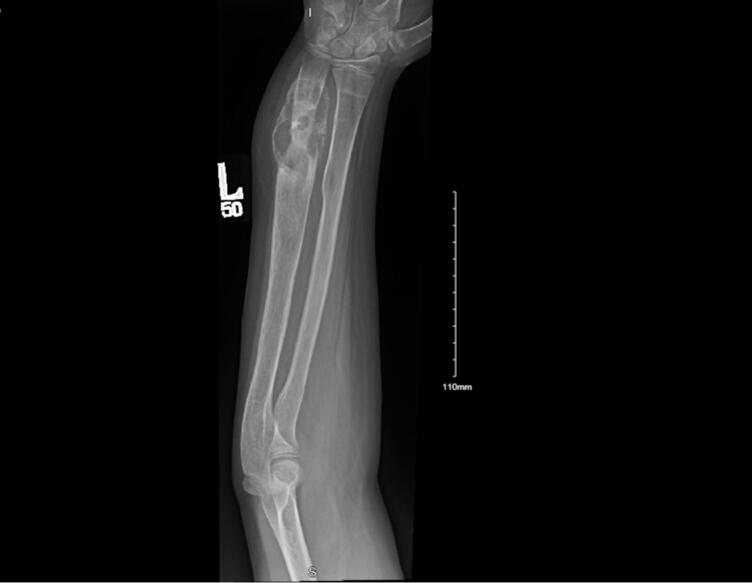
Radiograph obtained 1 year after the initial presentation; Anteroposterior view of the left forearm demonstrating a lytic lesion at the distal ulna.
